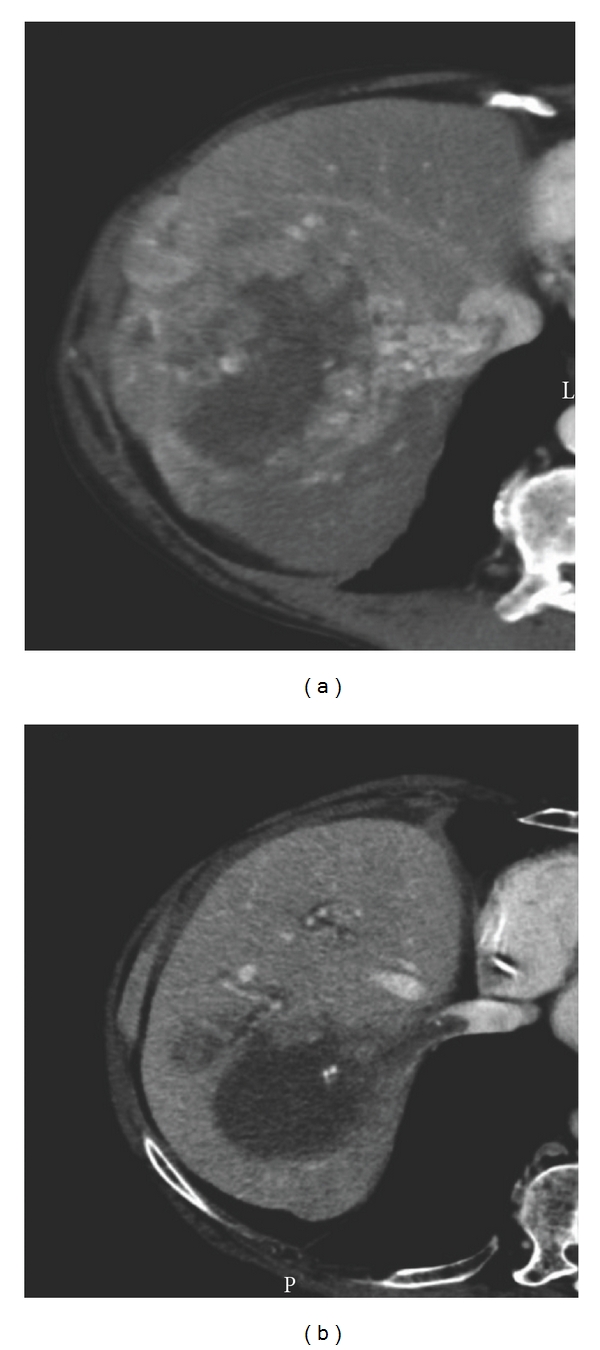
CT scan of patient 2.* (a) CT scan before treatment. (b) Reassessment CT scan after 9 months of treatment with sorafenib.
